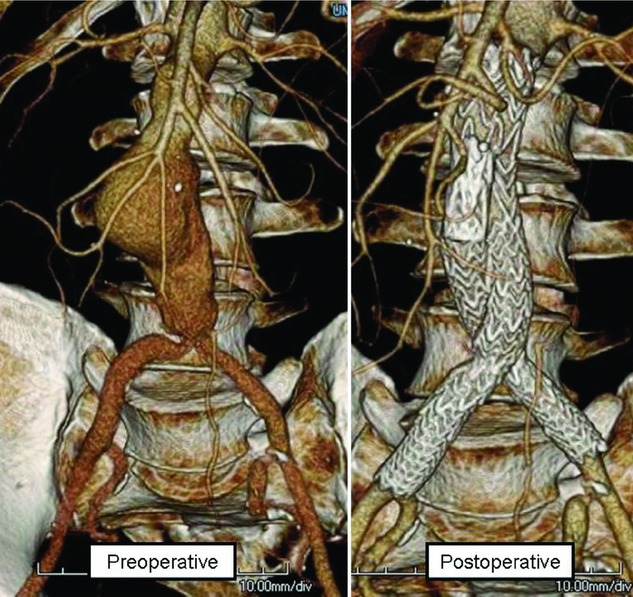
Endovascular repair of an infrarenal AAA demonstrating the preoperative and postoperative anatomy on CT scan with 3-dimensional reconstruction.
